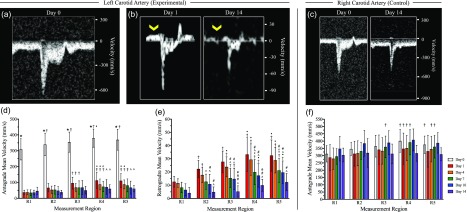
Hemodynamic characterization of LCA mean velocities between day 0 (a) and days 1 through 14 post-PCL (b) revealed a statistically significant decrease in antegrade blood flow (d) and an increase in retrograde blood flow (e). Caudal regions (R4 and R5) had statistically greater antegrade and retrograde velocities compared to cranial regions (R1 and R2) until day 7, as well as a steady decrease in overall velocity until day 14. Conversely, the contralateral control RCA revealed healthy pulsed wave Doppler (c) and antegrade flow velocities (f) over 14 days. Statistical significance: *p *<* *0.05 (★ = significance compared to all other days in the same region, † = significance compared to the same day in region 1, • = significance compared to the same day in region 2, ∧ = significance compared to day 1 in the same region, # = significance compared to day 4 in the same region, & = significance compared to day 7 in the same region, and ¥ = significance compared to day 10 in the same region).
